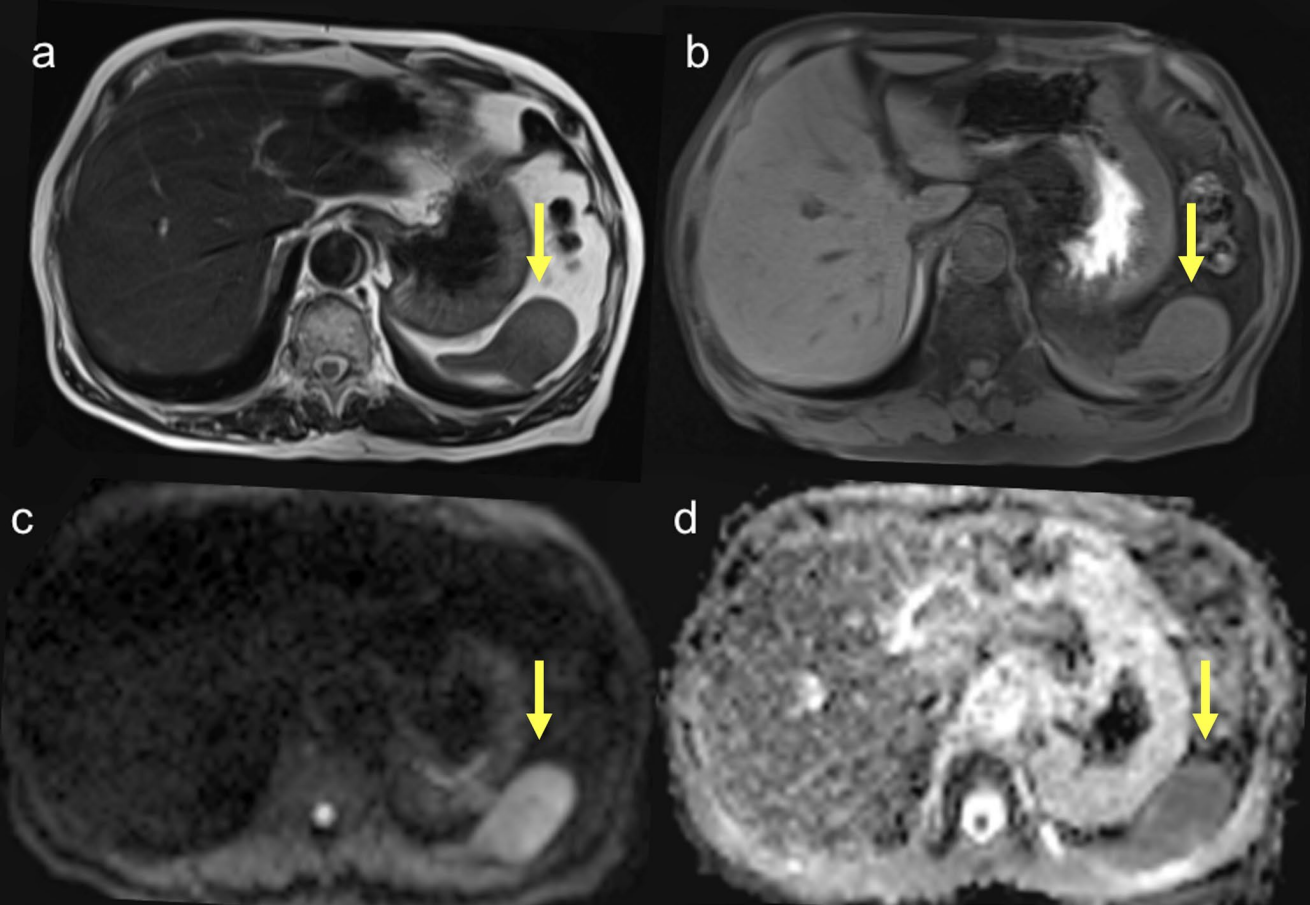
a, b T1- and T2-weighted images reveal a well-defined splenic mass, predominantly isointense to the splenic parenchyma, with linear and patchy hypointense foci. c DWI (b = 1000 s/mm^2^) reveals a high signal. d ADC map reveals a low value, consistent with restricted diffusion. DWI: diffusion-weighted imaging; ADC: apparent diffusion coefficient. The lesion is indicated by yellow arrows
